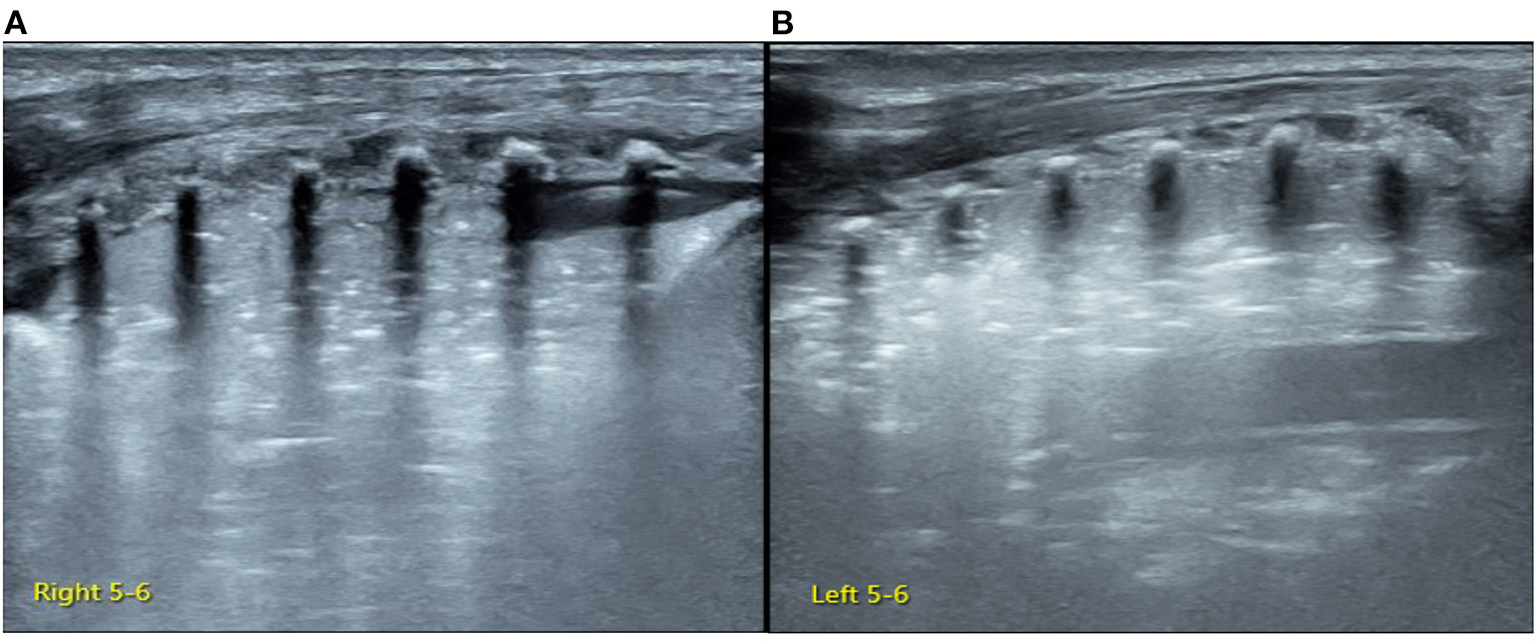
Lung ultrasonography (LUS) of the posterior chest. LUS examination of the right back close to the spine (A) and the left back (B) presents large consolidation with air bronchograms. Lung pulse is found under real-time ultrasound, which confirms the presence of large area atelectasis in bilateral lungs.
